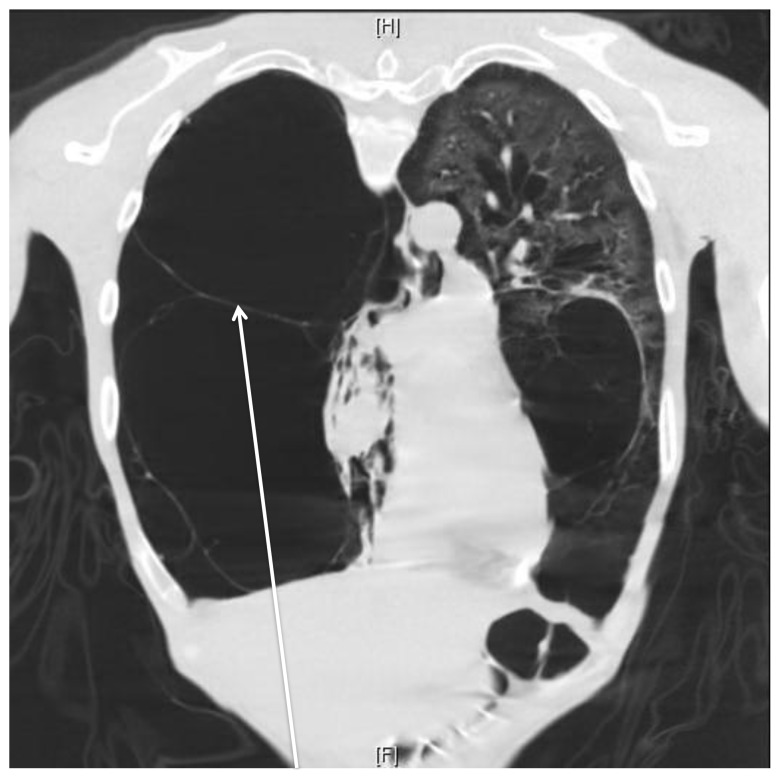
Coronal chest computed tomography demonstrating extensive bullous lung disease and no pneumothorax (arrow).
